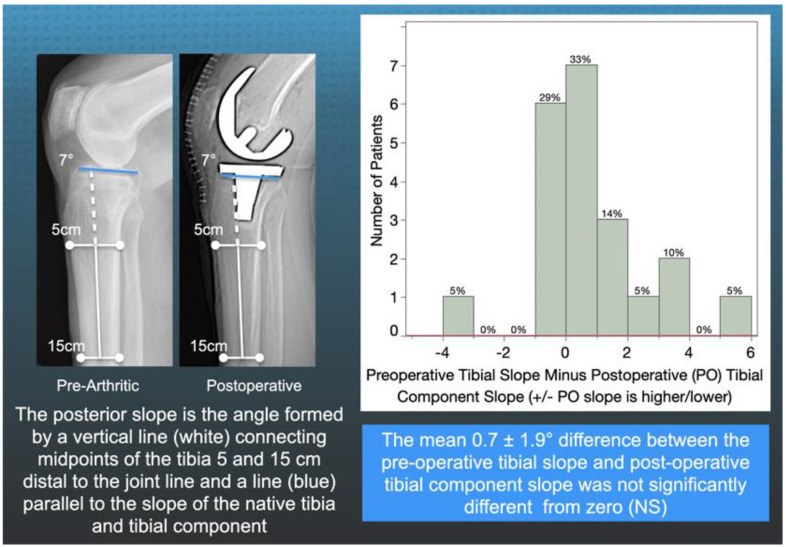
The figure shows the radiographic method for measuring the posterior slope of the preoperative tibia and postoperative tibial component (left) and the distribution of the difference in slope for the 21 patients as a measure of the reproducibility in setting the tibial component to the patient’s native slope using the angel wing (right).
